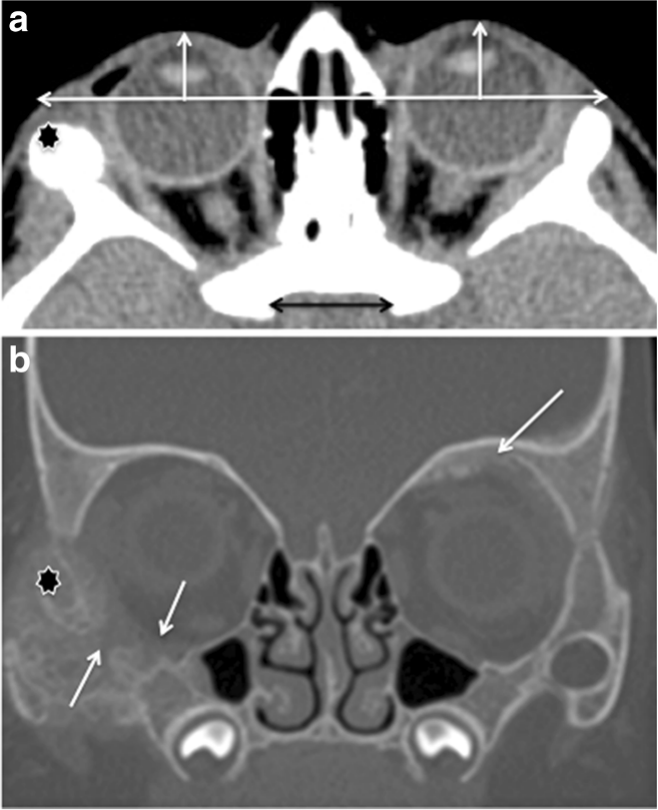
CT Scan showing enophthalmos of the right eye and the causal neoplastic process. a. CT cross-section showing a backwards displacement of the right eye comparatively to the left eye (Vertical white arrows). The baseline white bar does not sit on the lateral bony wall edge on the right side because of the osteolytic neoplastic process that compromises the integrity of the right zygomatic arch making it seem smaller but thicker and more heterogeneous (Black star, also in Figure
